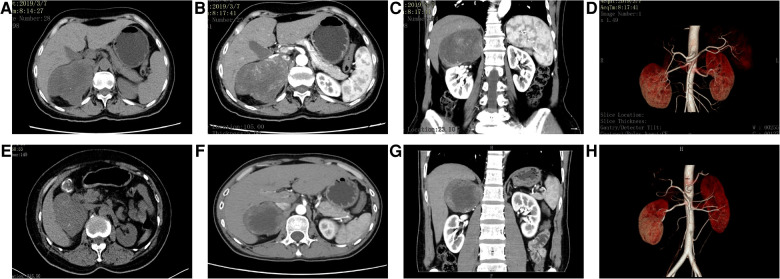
CT images of some patients. (A–D) Case 1: A mass-like mixed-density shadow was seen in the right adrenal area, and the boundary was still clear. The contrast-enhanced scan showed inhomogeneous progressive enhancement; the focus was mainly supplied by the small artery from the abdominal aorta, and the right renal artery was depressed. (E–H) Case 6: The mass density in the right adrenal area was slightly lower and the boundary was clear; the enhanced scan showed mild to moderate enhancement at the edge of the lesion, no enhancement in the central necrotic area, and compression changes in the liver and right kidney. The focus was mainly supplied by the right middle adrenal artery and inferior artery. Part of the blood vessels entered the mass, and the right renal artery moved downward under pressure.
